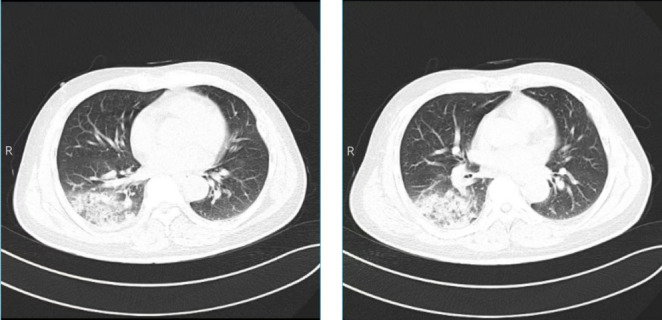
Representative chest CT image of the patient. Multiple patchy ground-glass opacities were visible in the lower lobe of the right lung, along with patchy consolidation, partial air-space consolidation, thickened interlobular septa, and air bronchogram. A small amount of pleural effusion was present. A small nodular shadow measuring approximately 4 mm in the longest diameter was visible in the lower lobe of the left lung.
